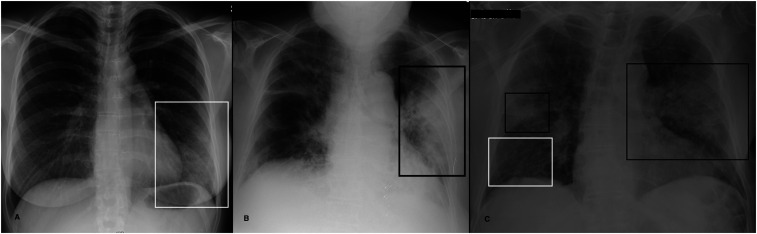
(A–C) Examples of chest X-ray abnormalities. (A) A 37-year-old woman with a 3-day fever, cough, and conjunctivitis. Chest X-ray shows a focal ground-glass opacity involving the lower field of the left lung (white rectangle). (B) An 86-year-old woman presented with dyspnea (SpO_2_ 88%), fever, and cough. Chest X-ray shows an area of consolidation in the middle-lower left fields (black rectangle). Ground-glass opacification (GGO) is recognizable in the right lung. (C) A 77-year-old man with a history of diabetes and arterial hypertension presented to the emergency department with a 7-day fever (38°C), dry cough, and dyspnea with low SpO_2_ (84%). Chest X-ray shows bilateral consolidations in middle lung fields (black rectangles). Bilateral areas of GGOs are also recognizable, particularly evident in the lower field of the right lung (white rectangle).
